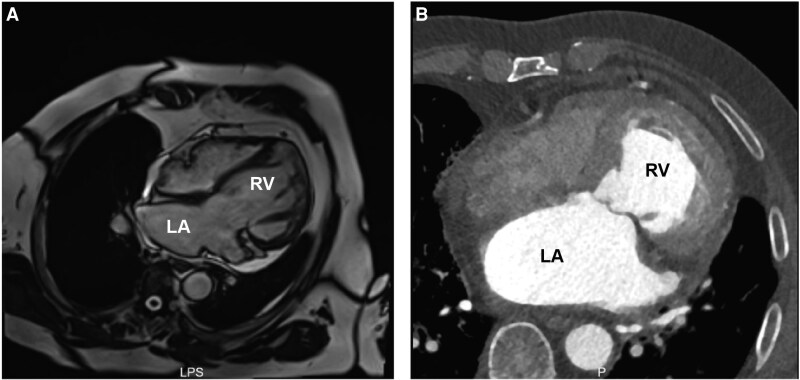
Four-chamber view on cardiac magnetic resonance (*A*) imaging and contrast-enhanced cardiac computed tomography (*B*). LA: left atrium; RV: right ventricle.
